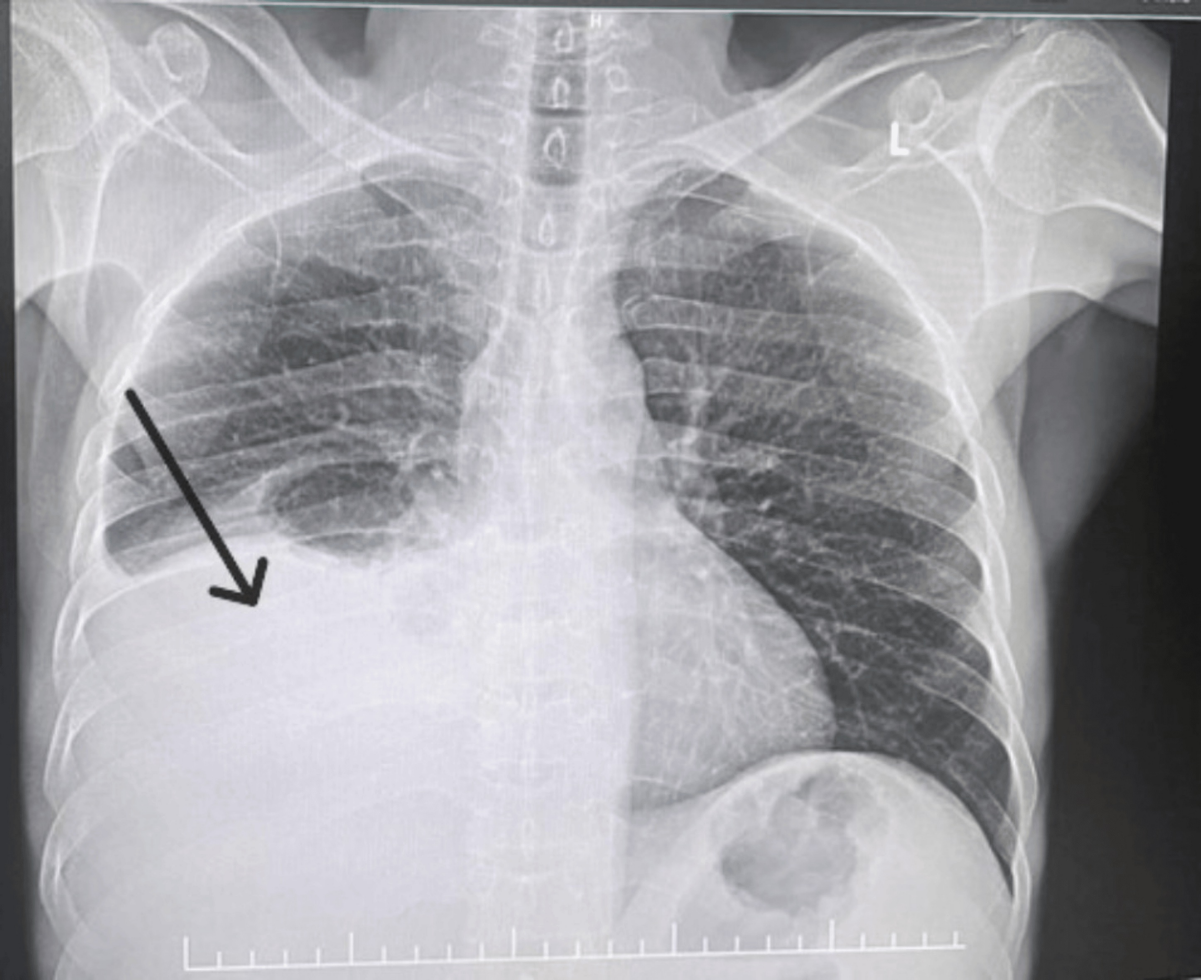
Chest X-ray showing right moderate pleural effusion (arrow) Blunting of the right costophrenic angle and homogeneous opacity of the lower and middle zones.
